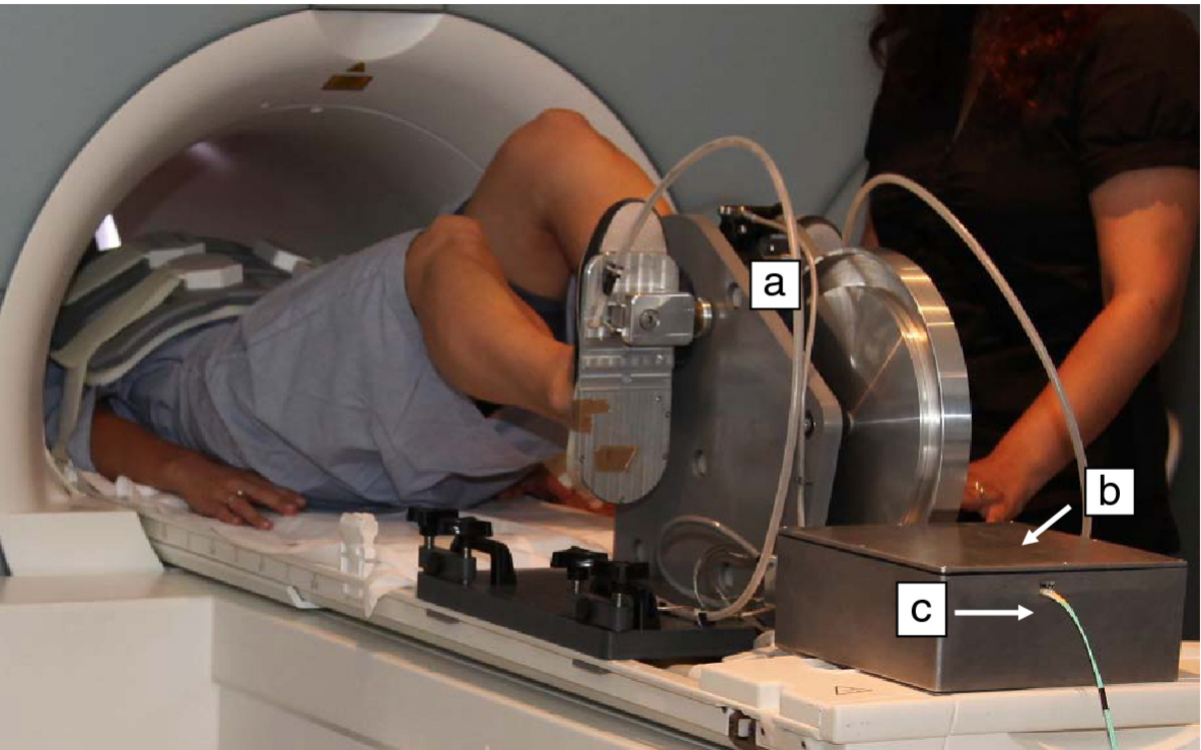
MRI cycle ergometer setting. Volunteer prep at MRI room with cycle ergometer (a) electronic box, (b) and optical fibre line, (c) before the MRI bed is moved to isocentre.
